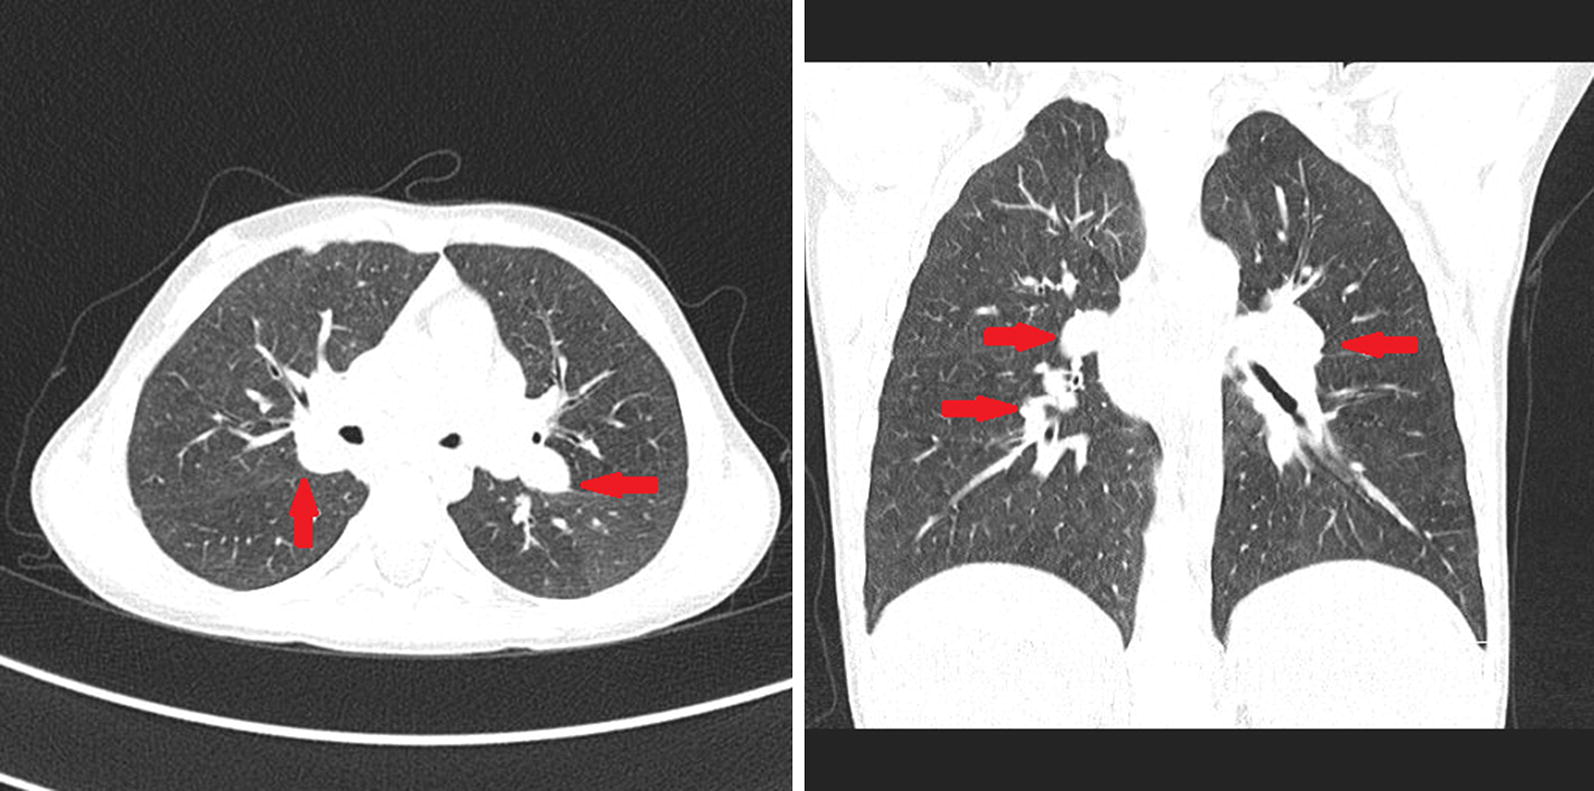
High-resolution computed tomography scan documenting severe lymphoproliferation within mediastinal lymph nodes of the 8-years-old boy with APDS 1
